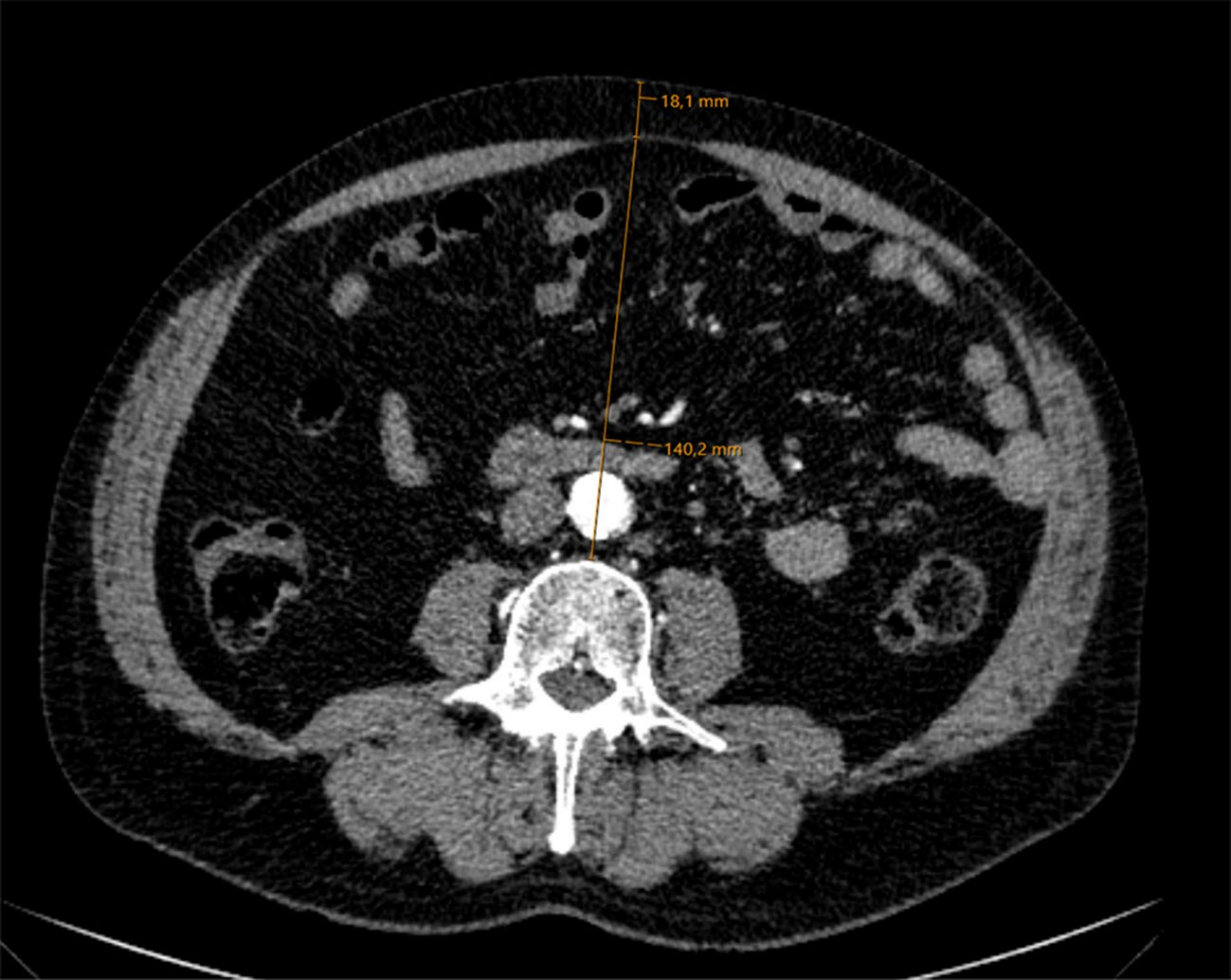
Contrast enhanced CT scan showing the measurement (orange line) of the visceral obesity from the ventral L3 vertebra to the linea alba and subcutaneous obesity from the linea alba to the skin.
